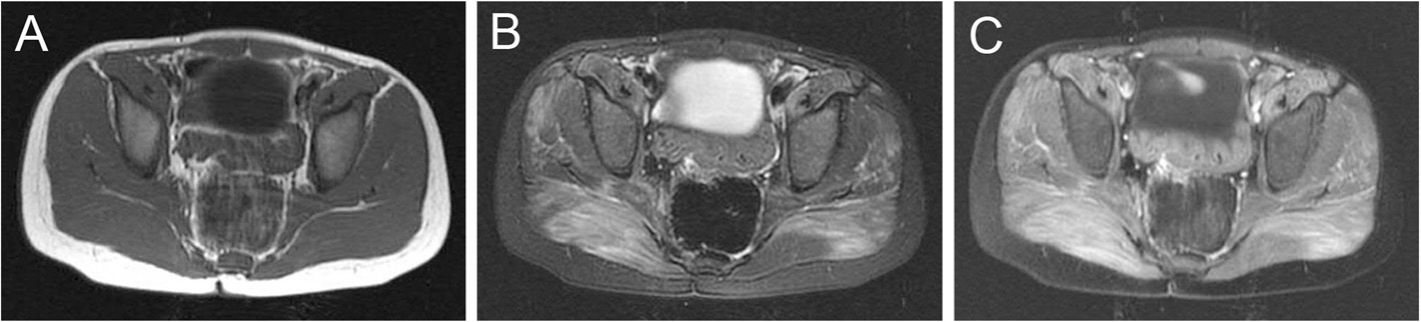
MRI of the gluteus maximus muscles demonstrating enhancement consistent with myopathy. (A) T1, (B) T2 fast spin echo (FSE), and (C) fat saturated T1 post gadolinium images demonstrate normal T1 signal and confluent increased T2 signal with associated gadolinium enhancement in the gluteus maximus muscles.
